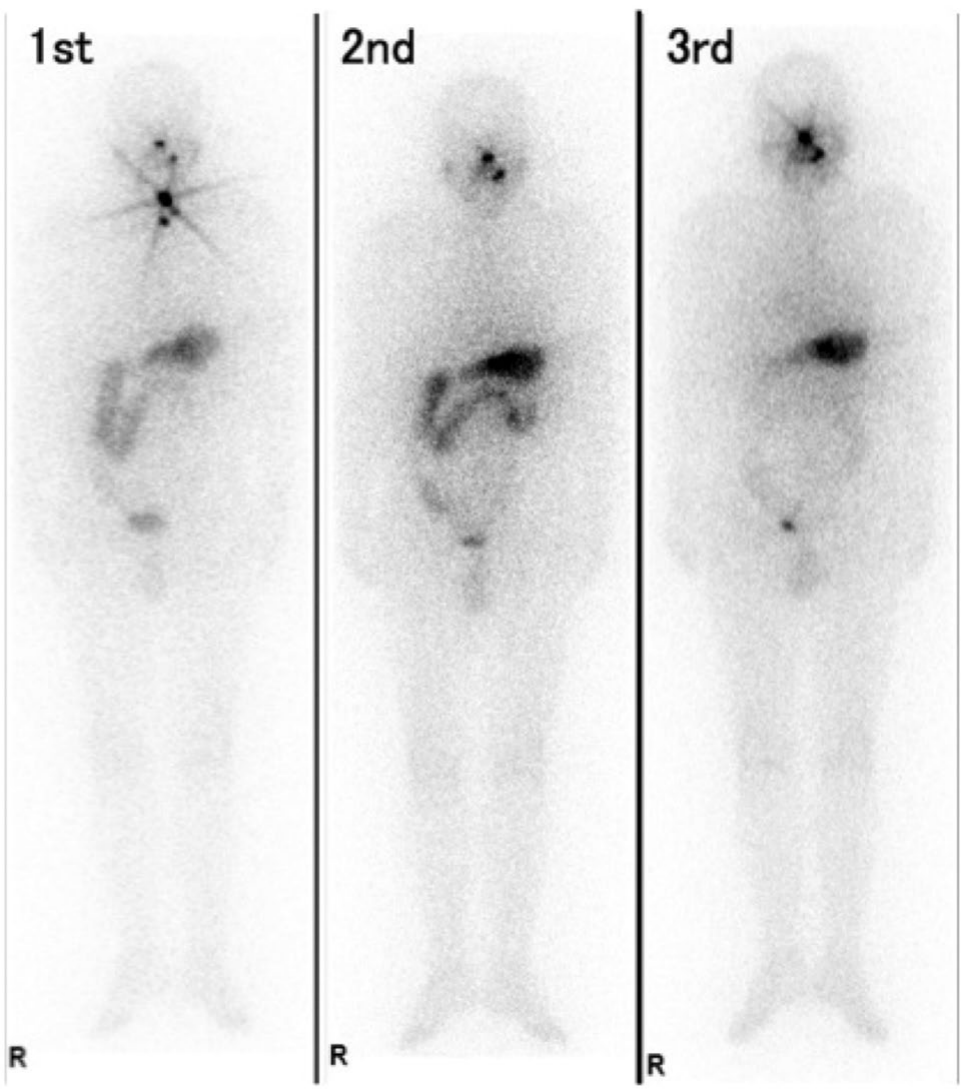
Frontal images of I-131 whole-body scintigraphy after radioactive iodine therapy in a man in his 60 s with lung metastases who received radioactive iodine therapy three times using thyroid hormone withdrawal method. The patient received 1.1 GBq of I-131 in the first RAIT, followed by 3.7 GBq of I-131 at the second and the third RAITs. He received the first and the second RAITs without laxatives. In his third RAIT, as he complained of constipation, he started using 24 mg of senna (stimulant laxative) 1 d before RAIT. As he did not receive quick relief from constipation, he continued taking senna for 3 d. Subsequently, he defecated thrice after RAIT. Colonic I-131 distribution was observed after the first two RAITs; in contrast, in the third RAIT, colonic I-131 distribution disappeared
